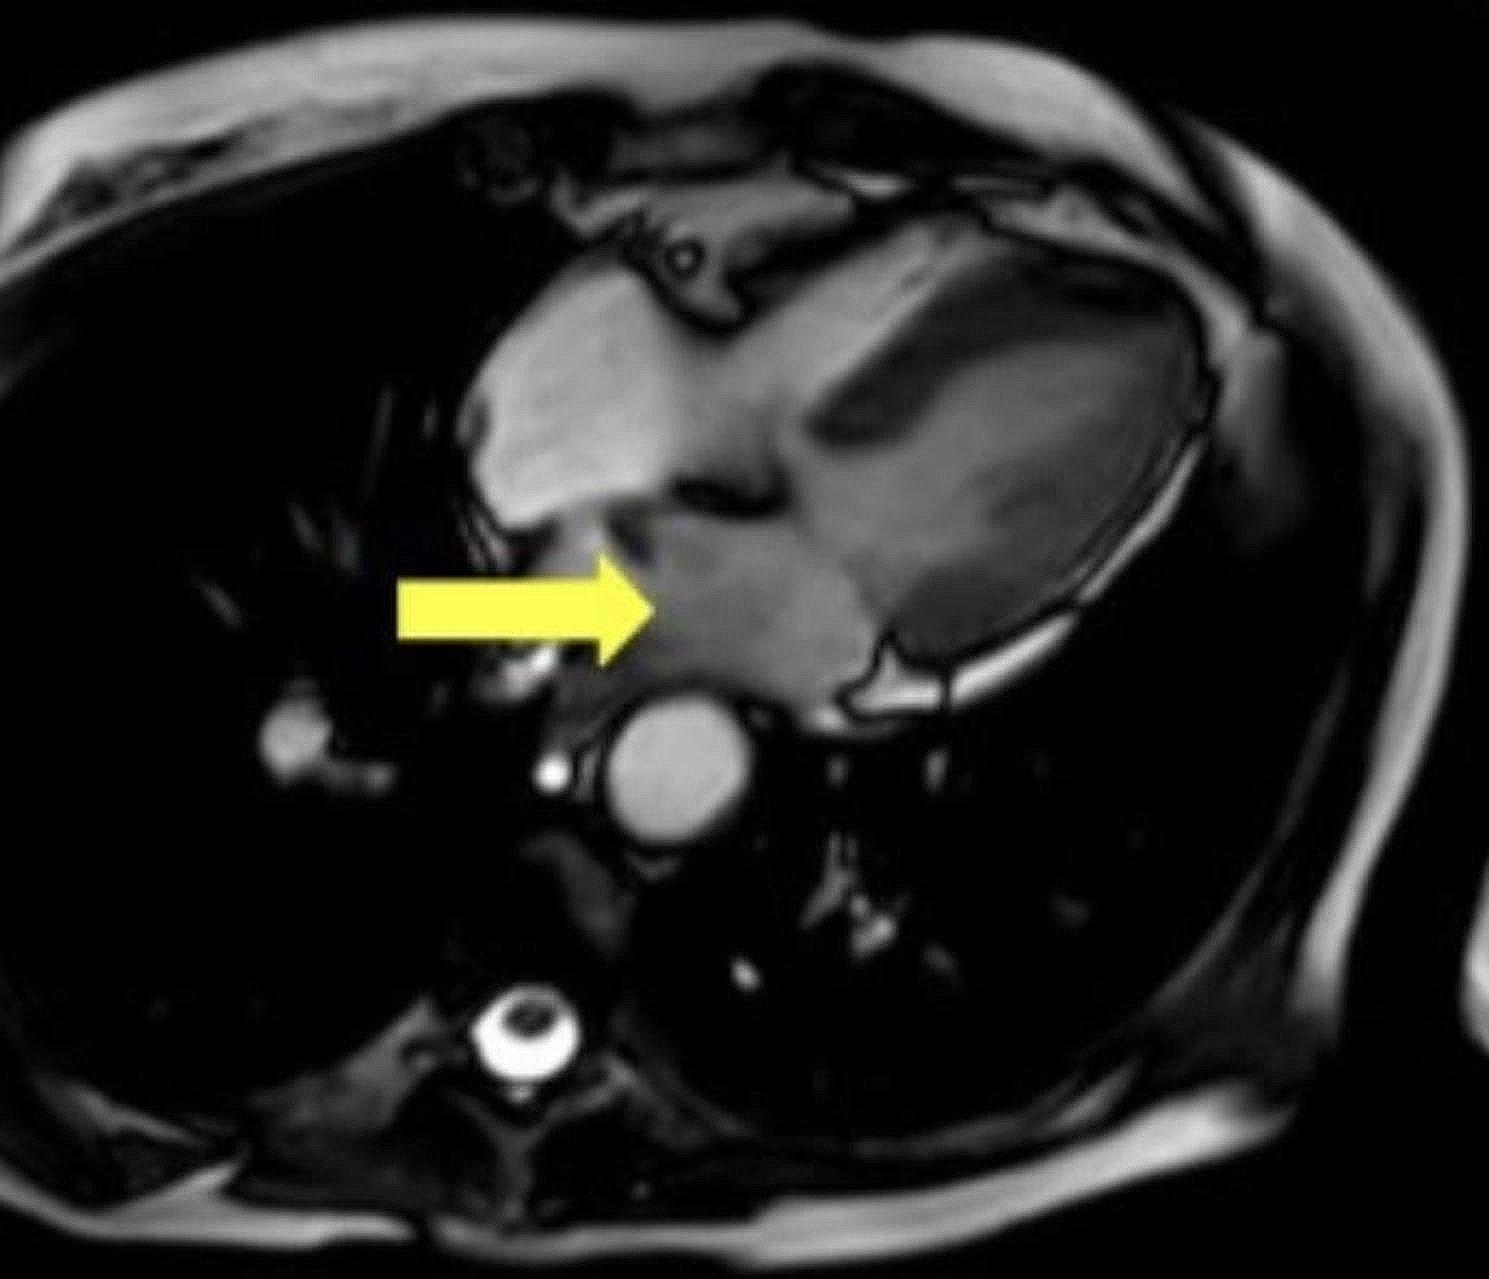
Cardiac magnetic resonance imaging (clip from a balanced steady state free precession [bSSFP] cine sequence) demonstrating a 2 cm X 1.1 cm hypointense lobulated mass lesion arising from the left atrium and interatrial septum at the level of fossa ovalis
